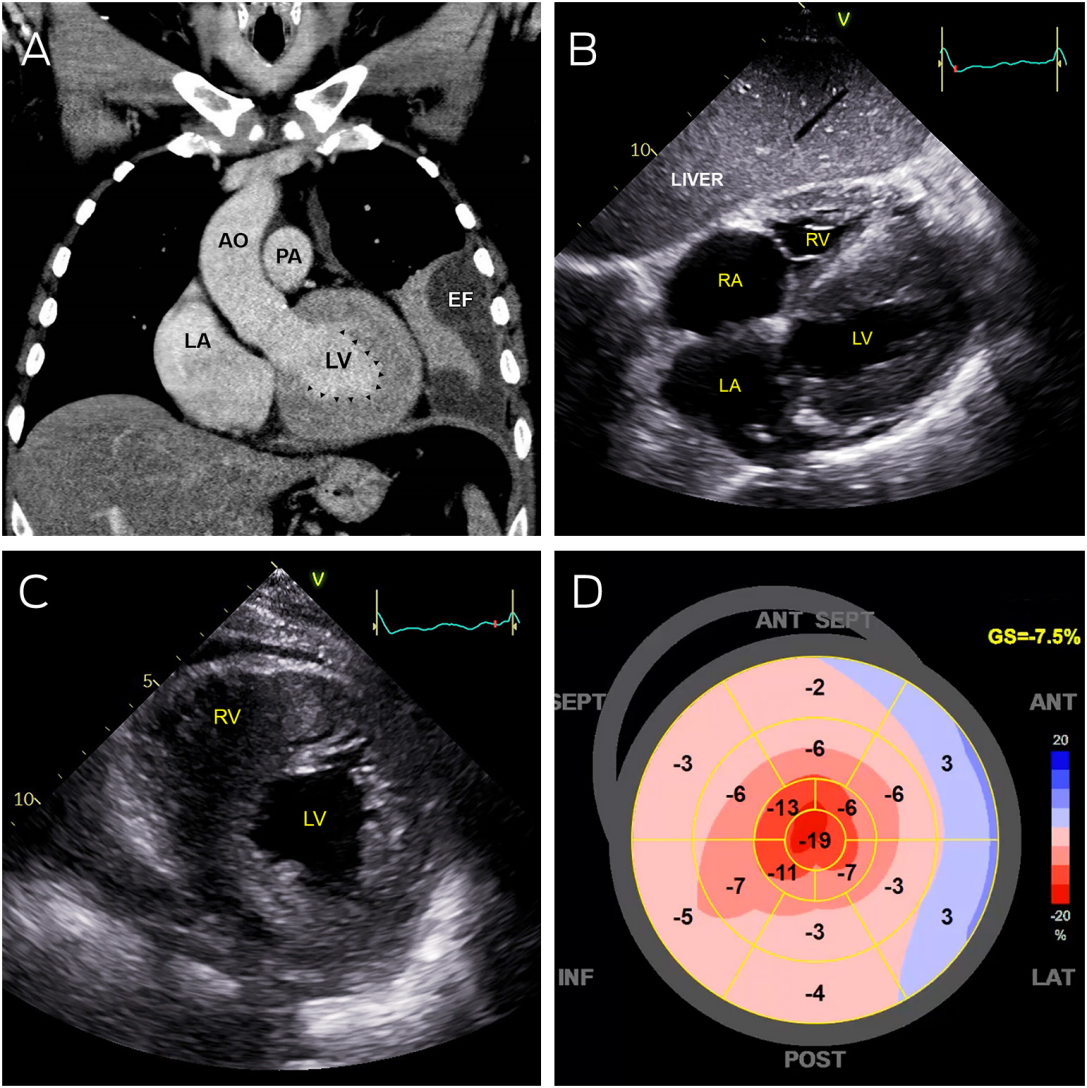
AO = aorta; EF = pleural effusion; LA = left atrium; LV = left ventricle; PA = pulmonary artery; RA = right atrium; RV = right ventricle. A: Coronal computed tomography showing severe left ventricular thickening without dilatation (arrowheads). B: Transthoracic echocardiography (subcostal view) showing severely thickened left and right ventricular walls, with severe bi‐atrial dilation. C: Transthoracic echocardiography (parasternal short axis view) showing severe increased left and right ventricular wall thickness. D: Strain map of the left ventricle showing systolic shortening of different walls of the left ventricle in a 2‐dimensional flattened image. Areas of bright red represent normal contraction while pink and blue areas represent hypocontractility. This strain map demonstrates apical preservation of contraction referred to as the cherry‐on‐top sign.
